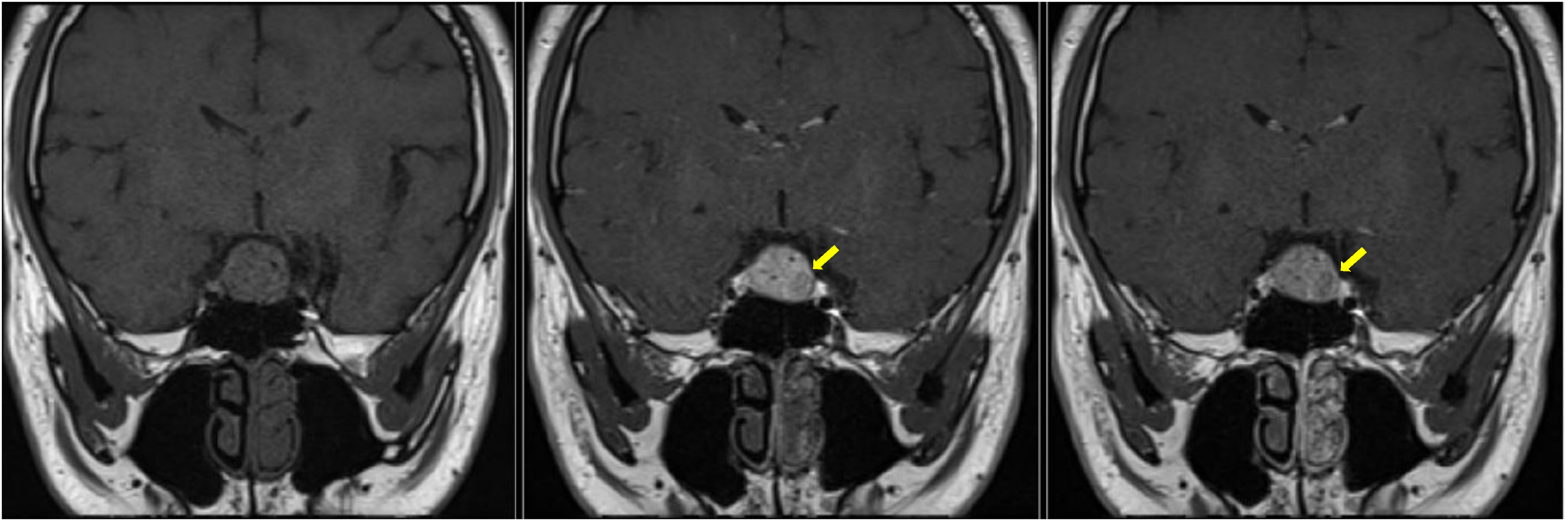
Dynamic contrast MRI (pre-operative). Yellow arrowhead, normal pituitary gland.
